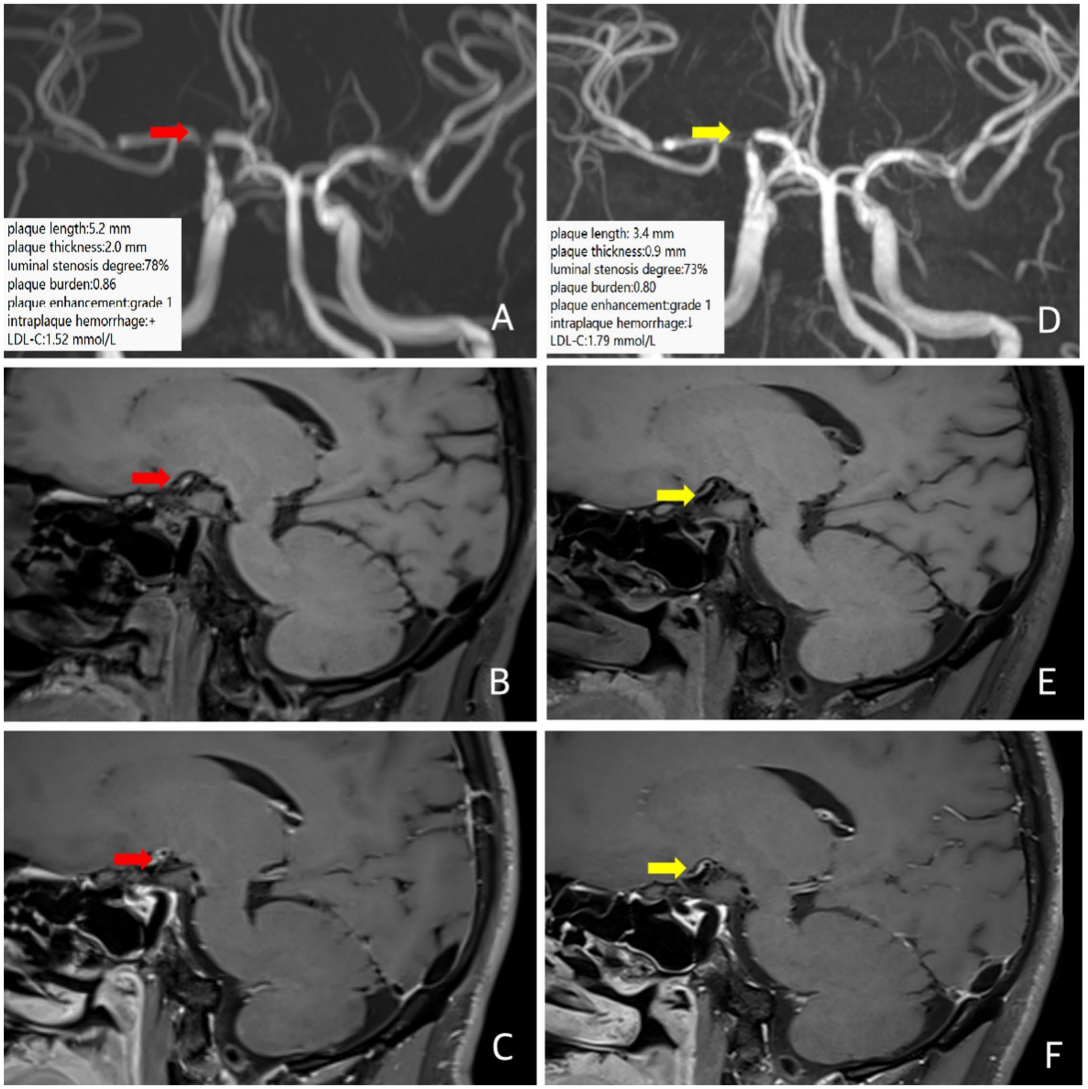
In case 3, the patient is at baseline (red arrow) and follow-up images 12 months later (yellow arrow). Three-dimensional time-of-flight cerebral magnetic resonance angiography showed significant stenosis of the right internal carotid artery communicating segment (A,D). Coronal T1 plain scan weighted imaging (B,E) and enhanced imaging (C,F) in whole brain magnetic resonance imaging using different inversion angles to optimize contrast showed atherosclerotic plaque was formed in the right internal carotid artery communicating segment, with obvious stenosis of the lumen, intraplaque hemorrhage (T1 signal significantly higher than the surrounding muscle signal) and obvious plaque enhancement. Follow-up intracranial VW-MRI showed a shorter plaque length and a lower plaque thickness than the baseline, a slight reduction in luminal stenosis and plaque burden, and a weakening of signals in intraplaque hemorrhage, suggesting that the plaque is receding and stabilizing. The LDL-C level is elevated.
